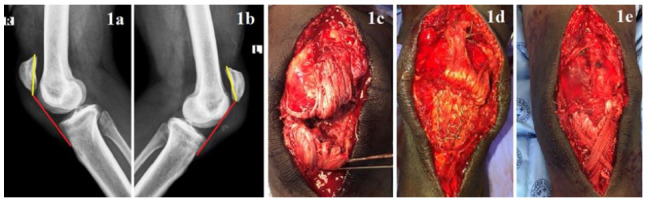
Lateral knee X-Rays showed increased Insall-Salvati Index (Left: 2.0, Right: 2.1).^[1]^
